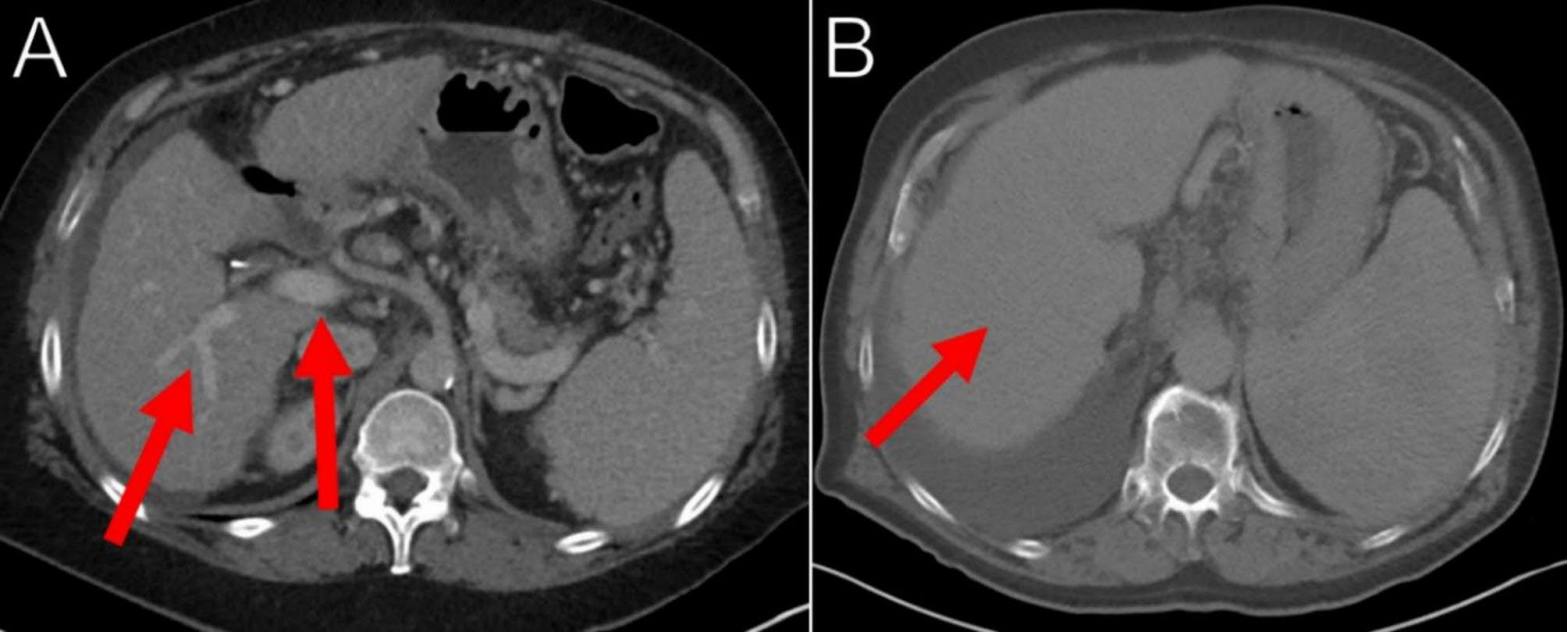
Variable Opacification of the Hepatic Vessels (A) Contrast enhanced CT in the portal venous phase displayed in the axial plane shows opacification of the hepatic vessels (arrows) making segmentation challenging but possible. (B) Contrast enhanced CT in the venous phase displayed in the axial plane demonstrates very poor opacification of the hepatic vessels (arrow) making segmentation and model creation impossible
